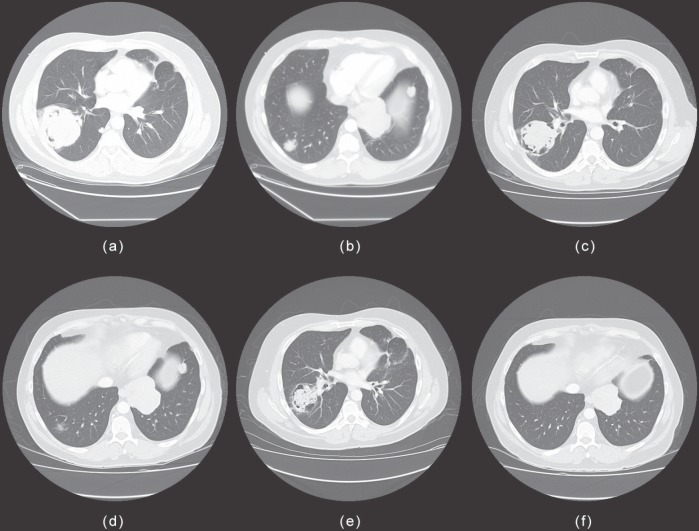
Chest CT scan of the patient treated with carboplatin and paclitaxel: the CT scan showed bilateral intrapulmonary metastases during initial workup with a 7.6 cm × 7.3 cm dominant mass in right lower lobe and a 7.4 cm × 8.2 cm dominant mass in left lower lobe (a,b); partial response after chemotherapy with carboplatin and paclitaxel (c,d); and on latest follow-up, the intrapulmonary masses and nodules had significantly reduced in size or remained stable (e,f). There was no evidence of any enlarging or new intrapulmonary lesions.
